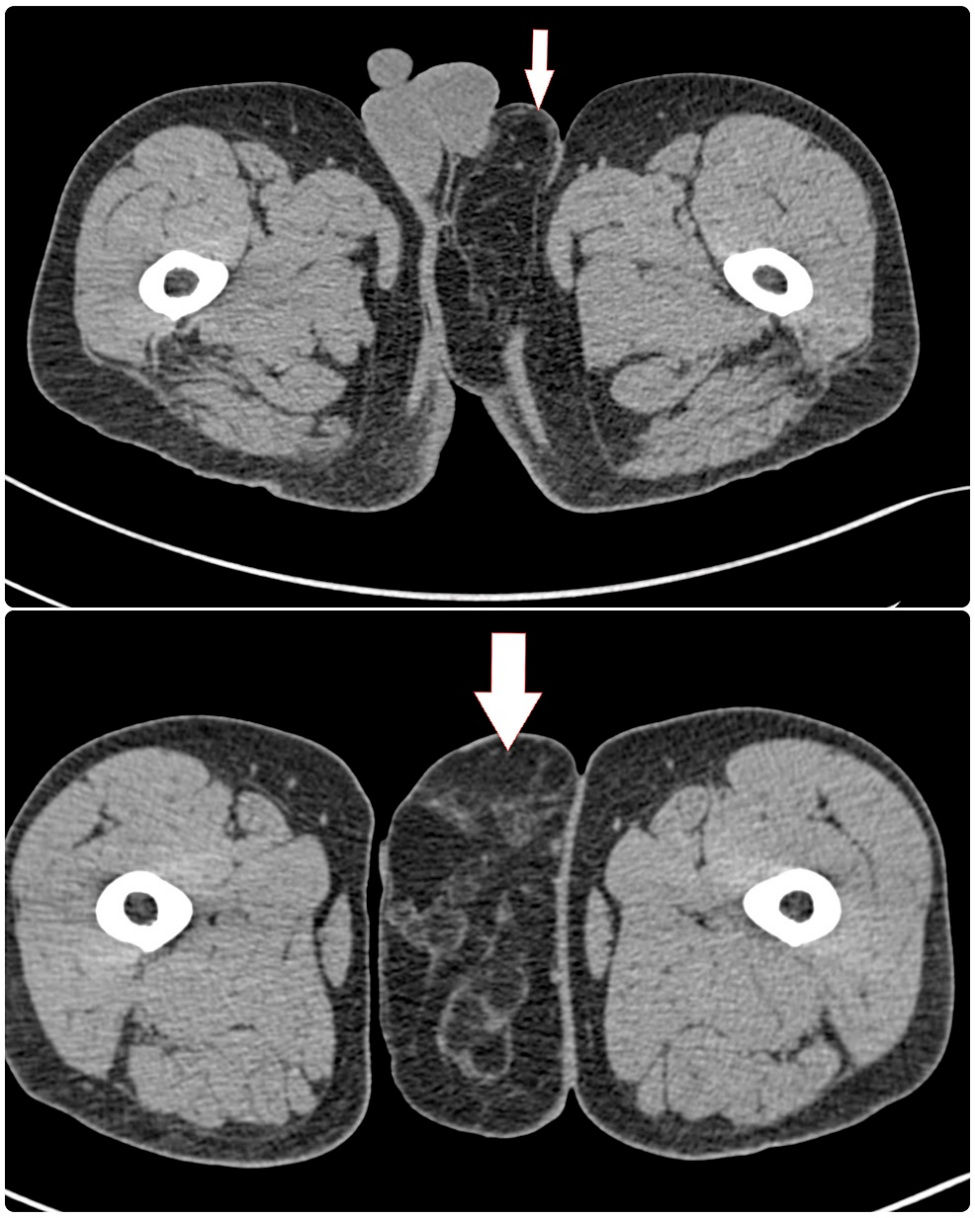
CECT images showing the origin of para-scrotal liposarcoma CECT: contrast-enhanced computed tomography
